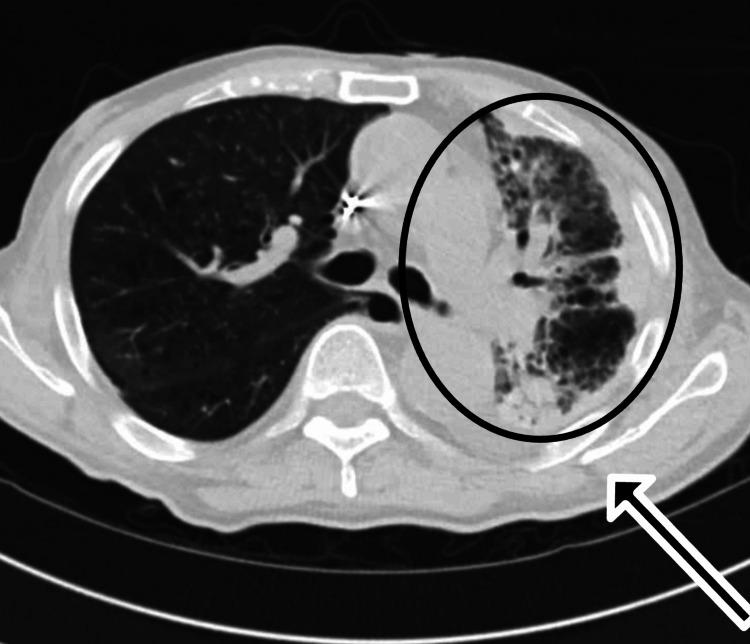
Computed tomography (CT) scan of chest axial view showing extensive pleural thickening, peripheral opacities, and fibrotic changes throughout the left lung. This pronounced asymmetry is unusual for amiodarone-induced pulmonary toxicity and underscores the diagnostic challenge posed by unilateral disease. In the absence of infectious, autoimmune, or malignant etiologies, these findings supported the diagnosis of amiodarone-related lung injury.
